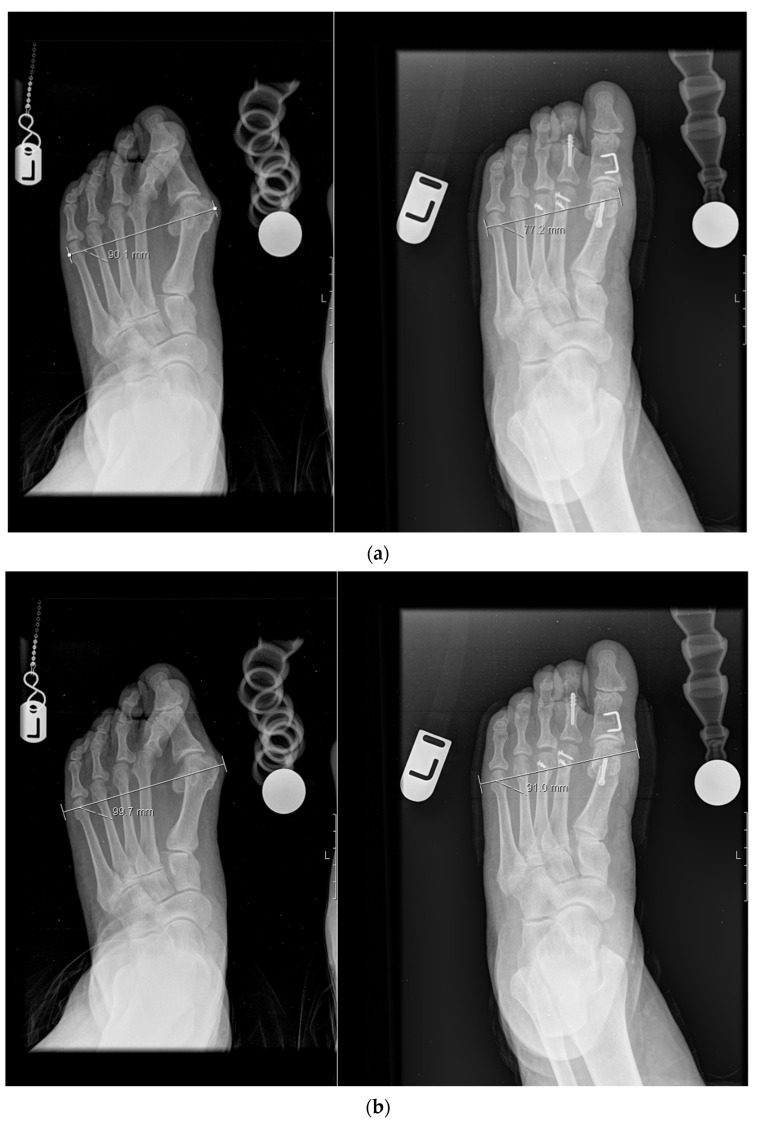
Pre and postoperative measurements of (a) BW and (b) STW on standing calibrated X-rays.
